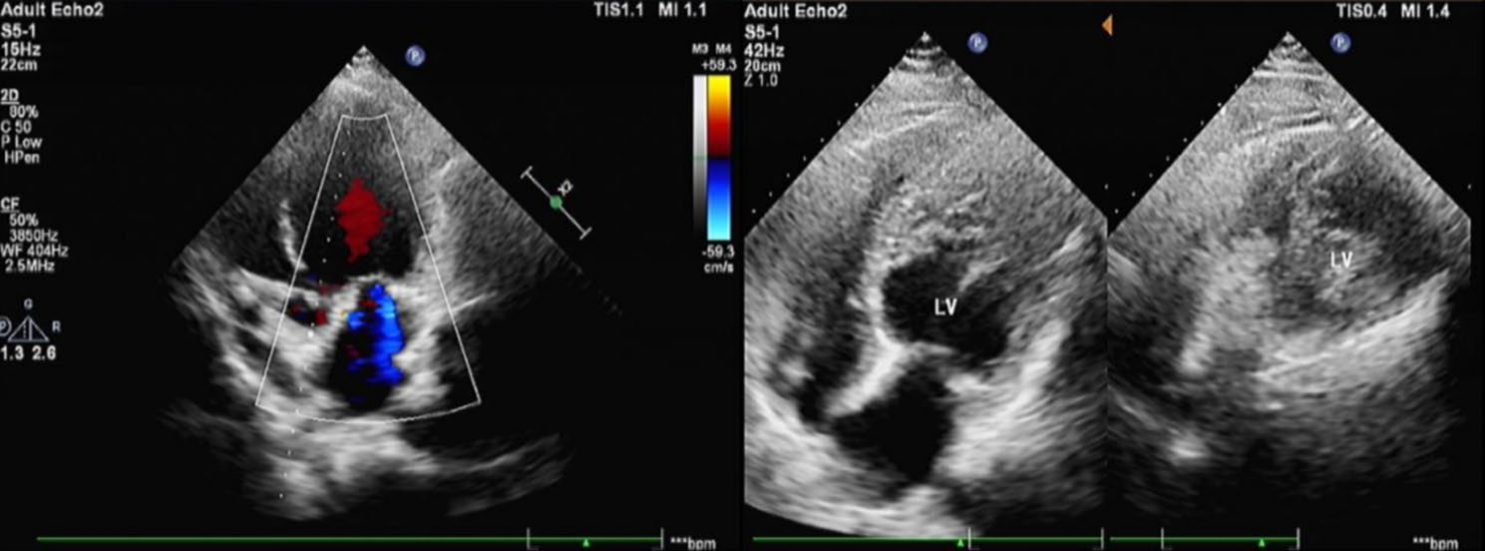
Echocardiography showed increased myofascicular echoes in the posterior lateral wall and apical region of the left ventricle, and the myocardium was loose in texture, forming crypt-like changes
